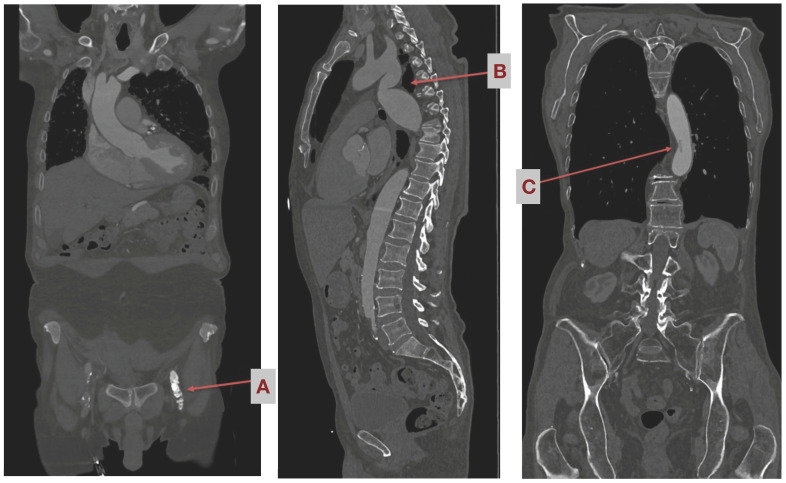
Incidental finding with contra-indications for femoral cannulation and retrograde blood flow as seen in preoperative CT angiography scan. (A) Occlusion of common femoral artery, (B) Stenosis of aortic isthmus, (C) Thrombus in the abdominal aorta.
